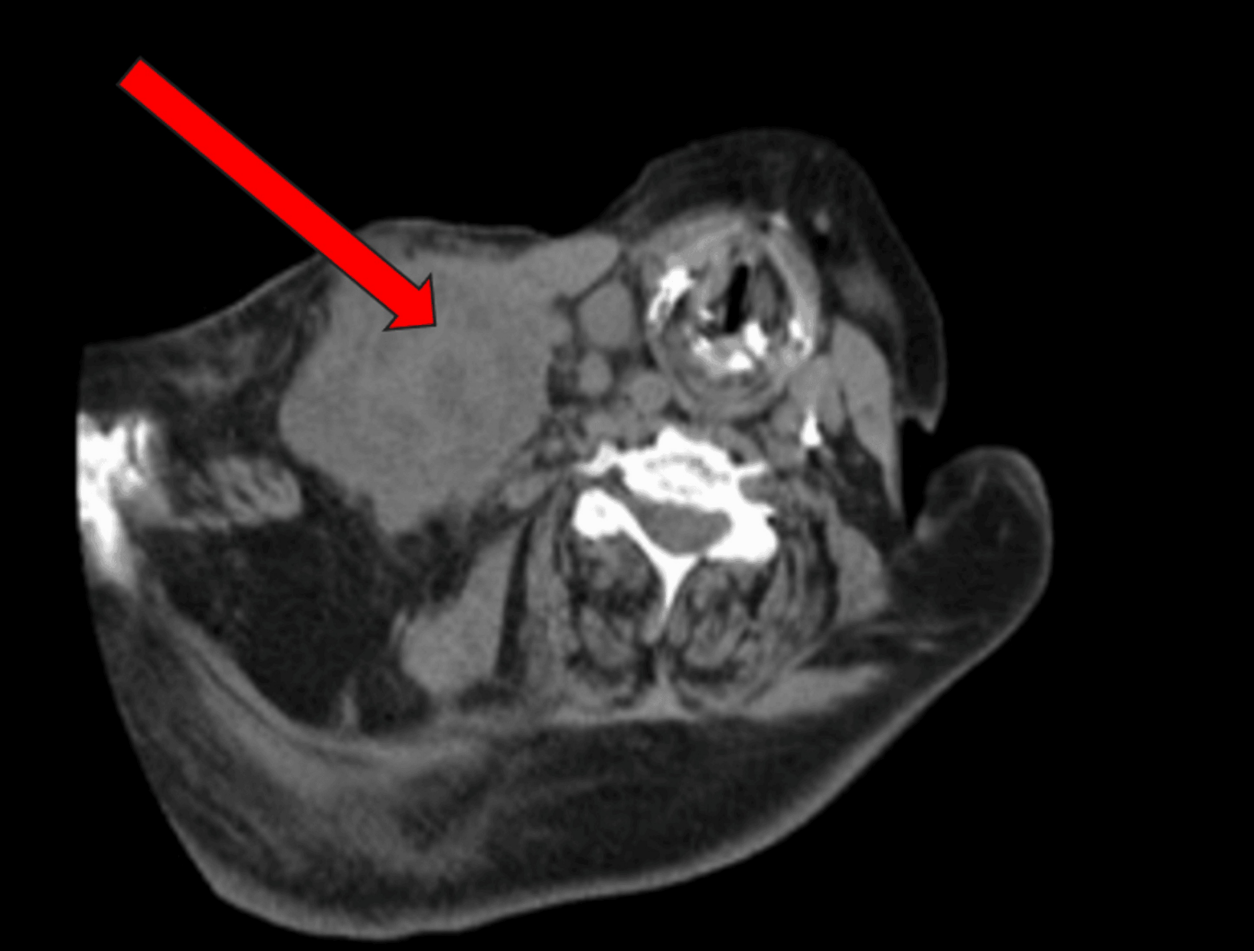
CT neck without contrast The red arrow indicates the interval enlargement of the right paraclavicular neck mass, which extends into the infraclavicular region adjacent to the right subclavian vein.
